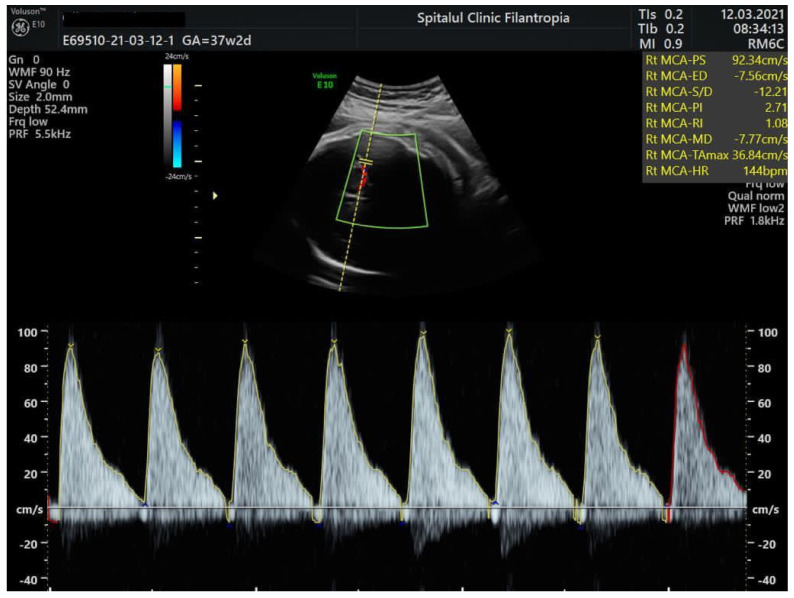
Doppler studies of mean cerebral artery (MCA) in a term baby showing increased peak systolic velocity (PSV) and absent end-diastolic flow.
